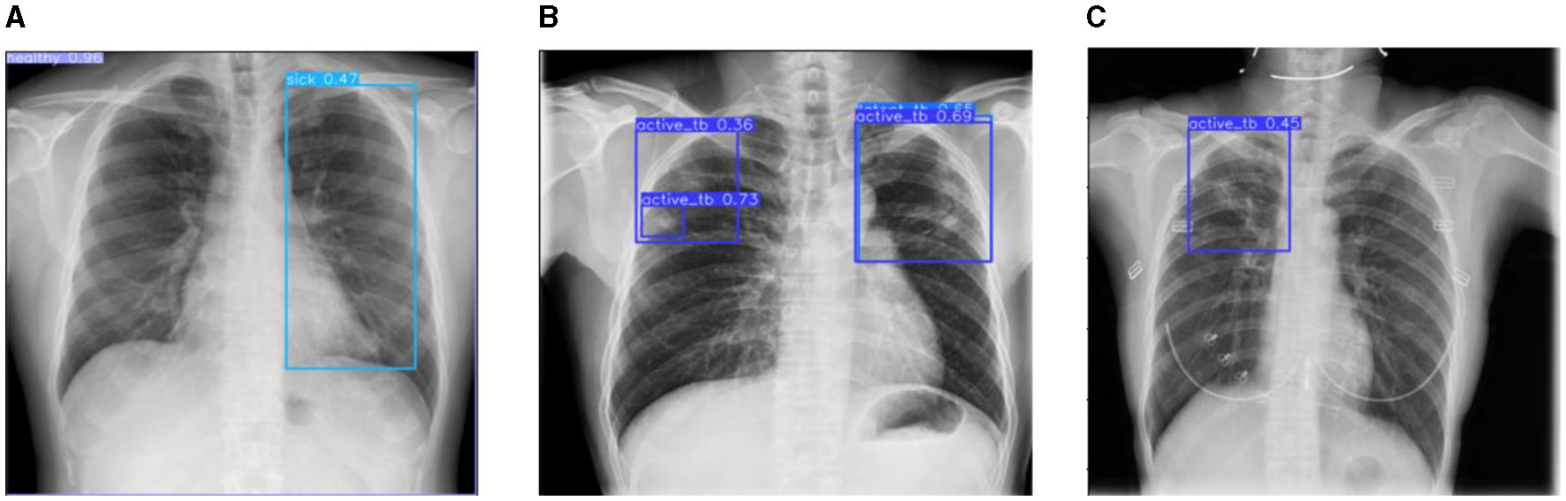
Prediction results of existing YOLOv8 model. (A) X-ray with both healthy and sick regions. (B, C) X-ray with active TB and latent TB.
